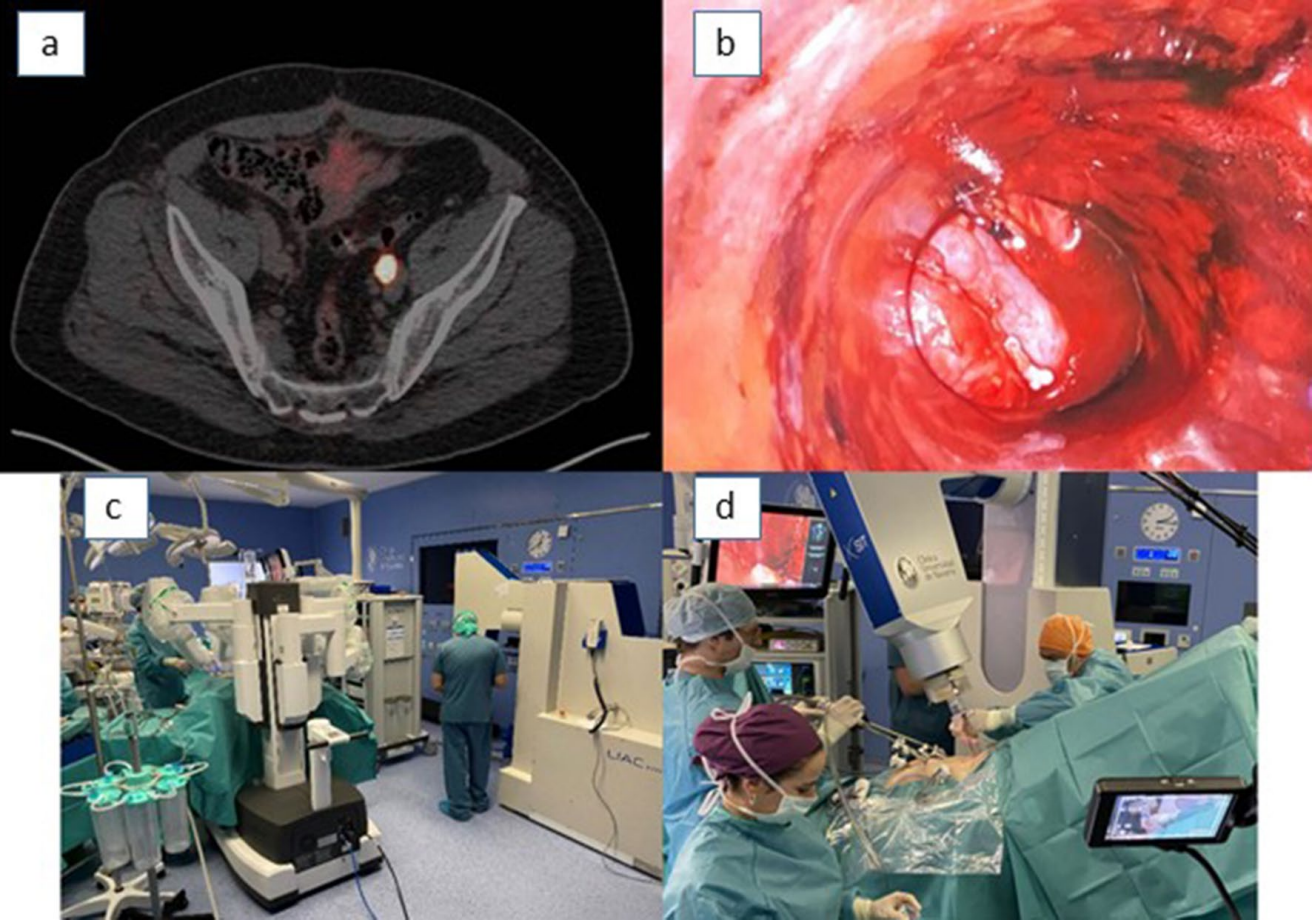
View of the IOeRT procedure during robotic surgery. a PET-PSMA showing a single nodal recurrence at the common iliac vessels, 2.7 cm in maximal diameter; b after nodal removal, the superior and inferior limit of the nodal bed is marked with fiducials seen via the interior of the applicator (4 cm in diameter), while intrapelvic normal tissues uninvolved by cancer are displaced from the electron beam (ureter, bladder, rectum, small bowel); c operating room view with miniaturized technologies present at the time of the surgical procedure: left da Vinci robotic system, right IOeRT linear accelerator; d docking procedure sequential to robotic resection, and IOeRT applicator positioning under video control while the pneumoperitoneum is maintained
